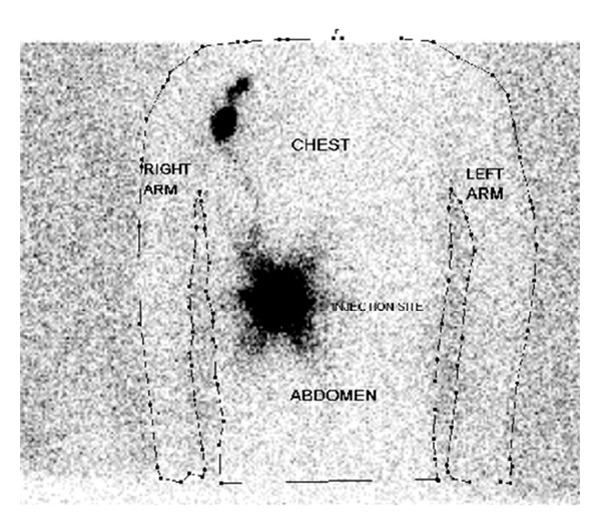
Lymphoscintigraphy demonstrating uptake in the right axilla.
